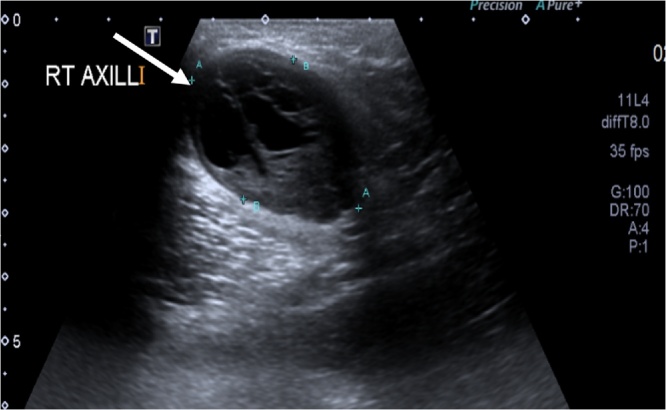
Ultrasound of the right axillary area showing a mass with cystic degeneration.
